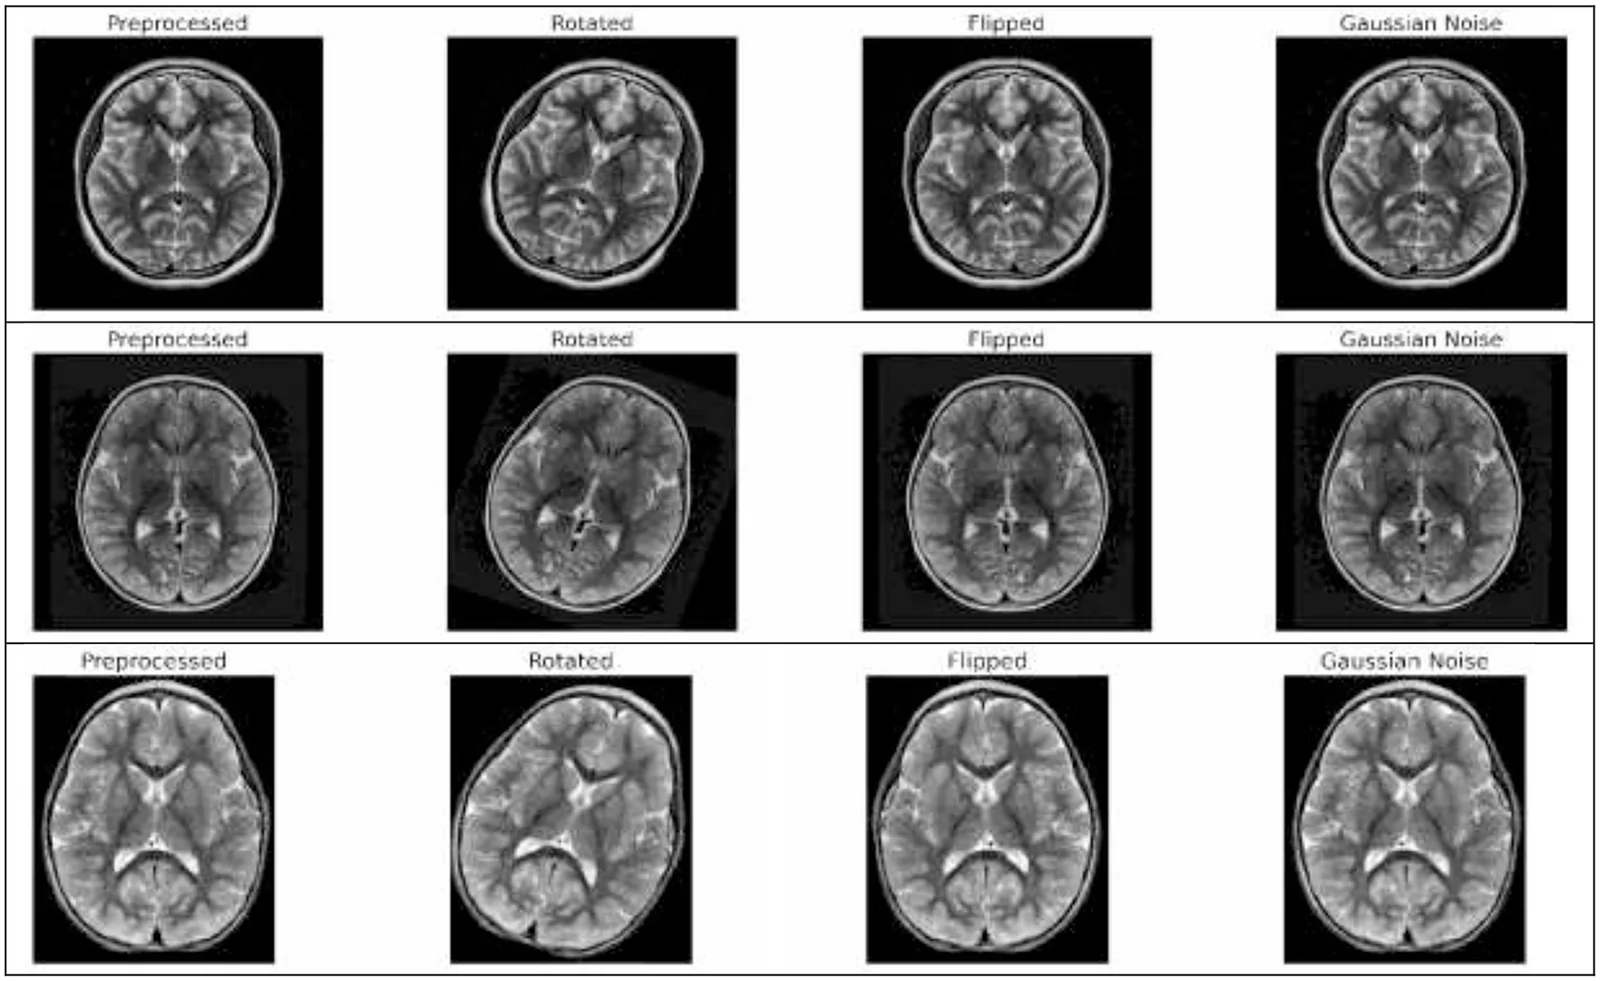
Augmented images of the CT dataset.
